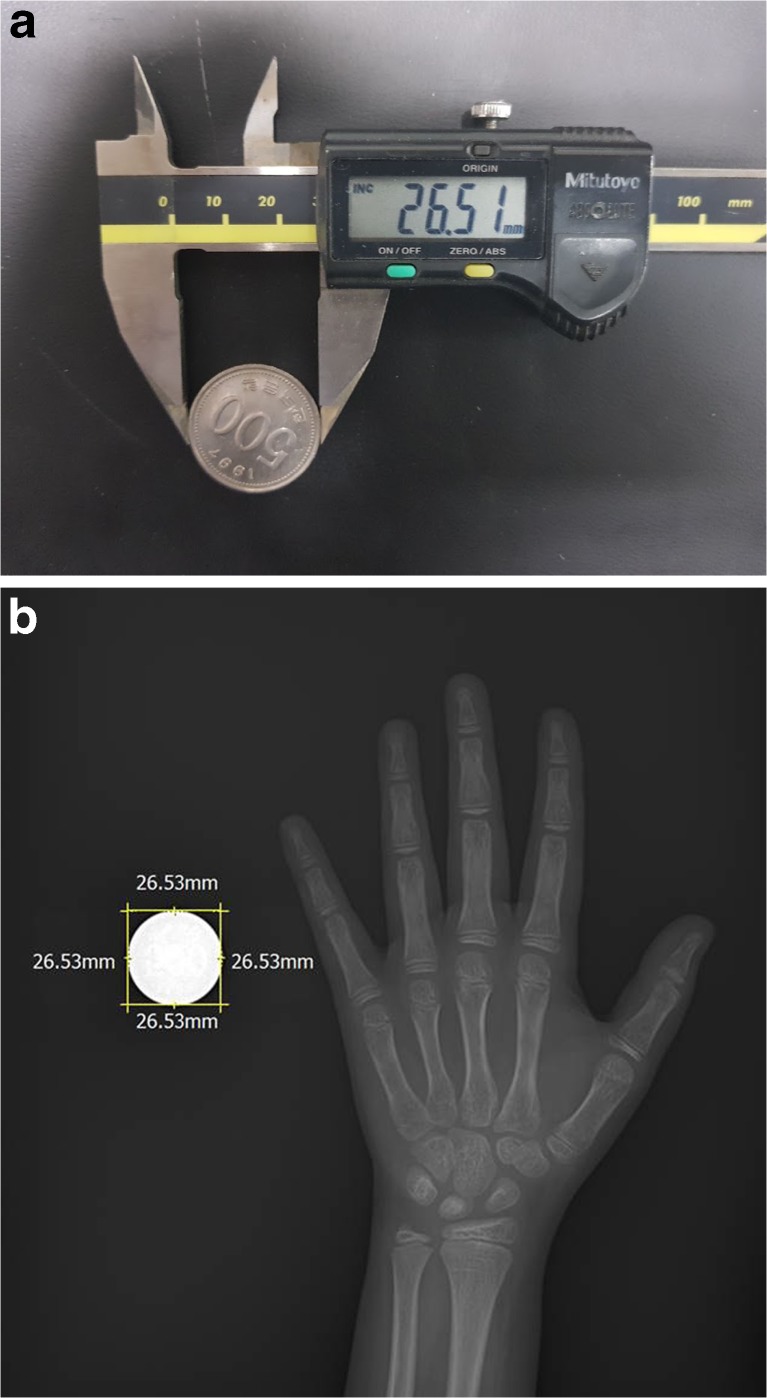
(a) The diameter of a coin measured using a digital micrometre was 26.51 mm. (b) The diameter of the coin on a digital radiograph was measured as 26.53 mm on a Picture Archiving and Communication System (PACS)
